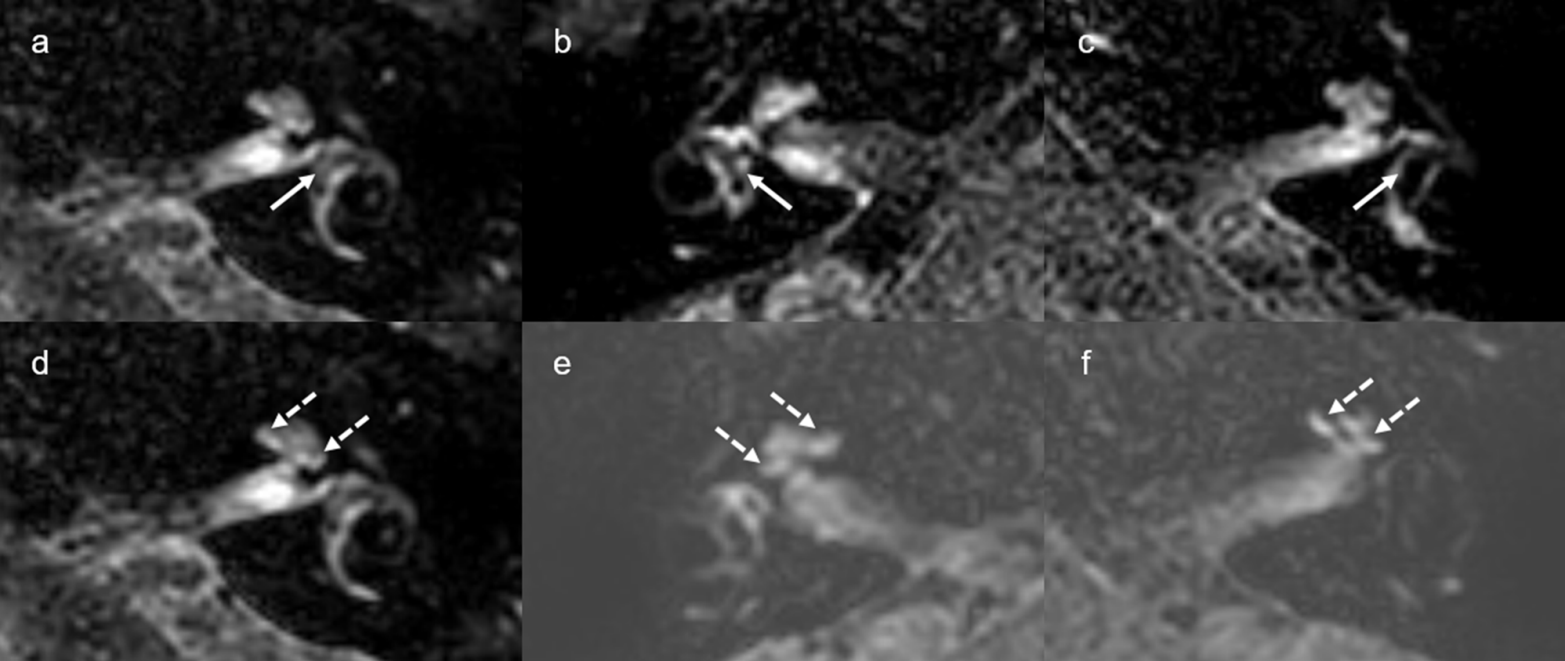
Delayed 3D FLAIR MRI of vestibular endolymphatic hydrops graded none (a), grade I (b), grade II (c), and cochlear endolymphatic hydrops graded none (d), grade I (e), grade II (f). Vestibular endolymphatic hydrops are pointed with white arrows, and cochlear endolymphatic hydrops are pointes with white dotted arrows
